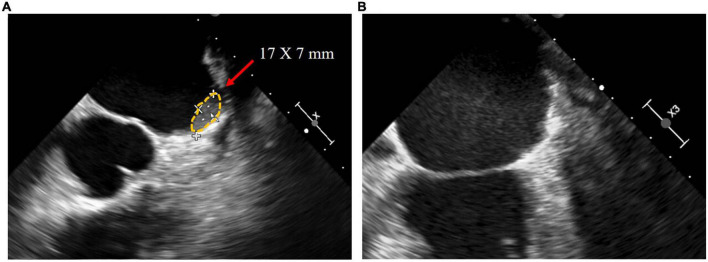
In the transesophageal echocardiography images of patient B, (A) DRT of 17*7 mm was detected 14 months postoperatively, and (B) DRT dissolved after 5 months of 2.5 mg warfarin once daily.
